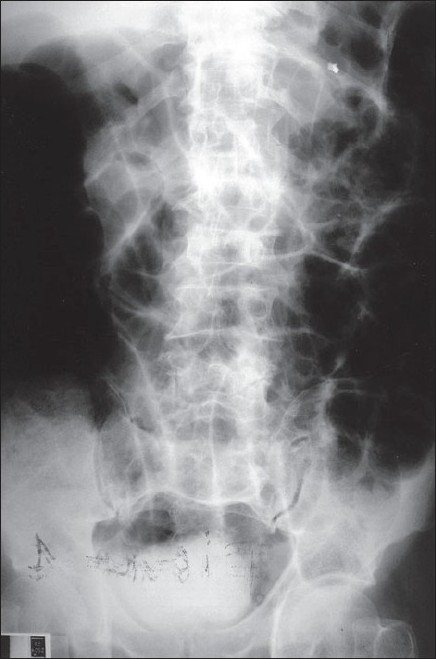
Flat abdominal X-ray. Intestinal obstruction
